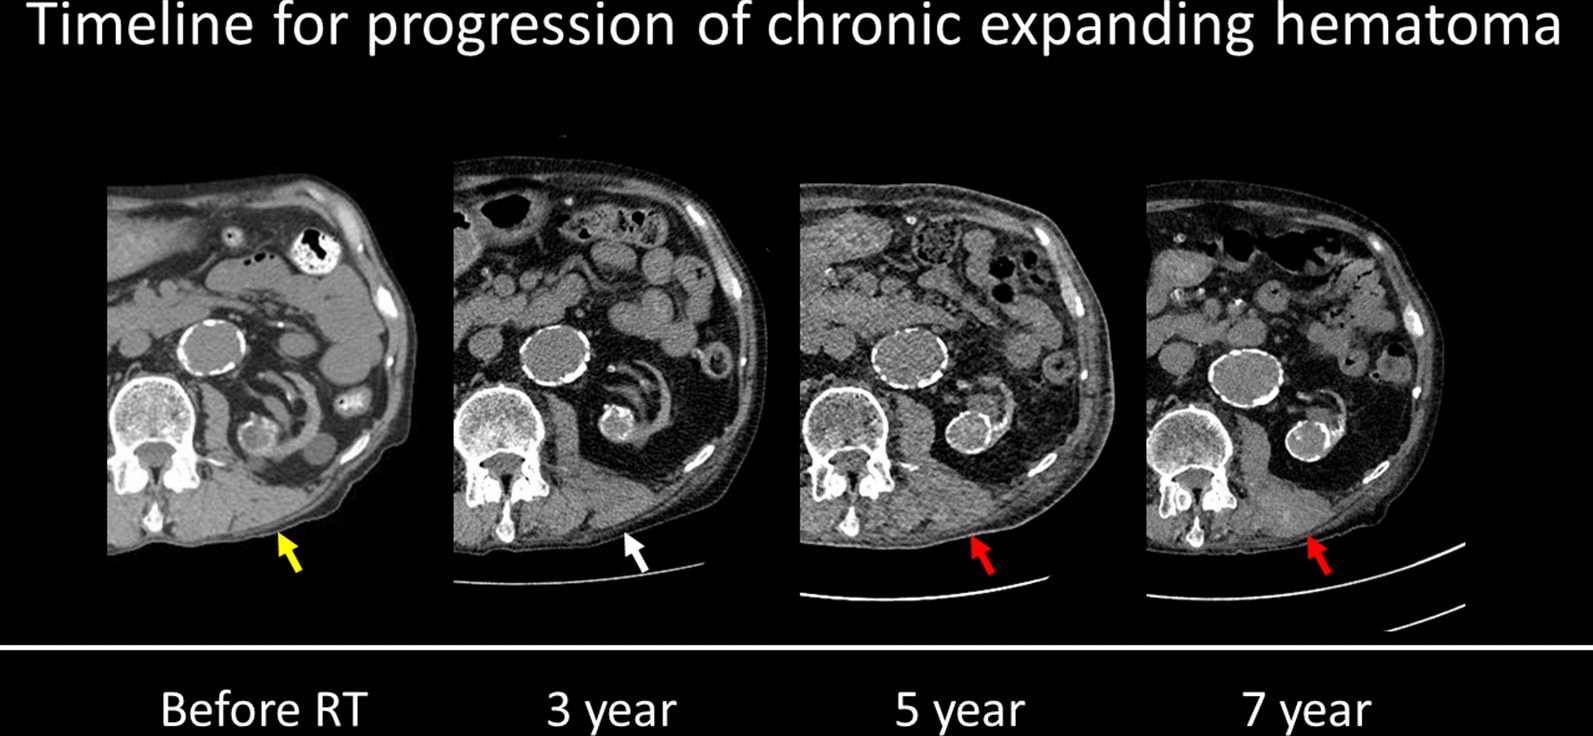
Timeline for progression of the chronic expanding hematoma on computed tomography (yellow arrow). Before SBRT, the left erector spinae muscle was normal. The muscle gradually became atrophic 3 years after SBRT (white arrow). Finally, the muscle was swollen between 5 and 7 years after SBRT (red arrow).
